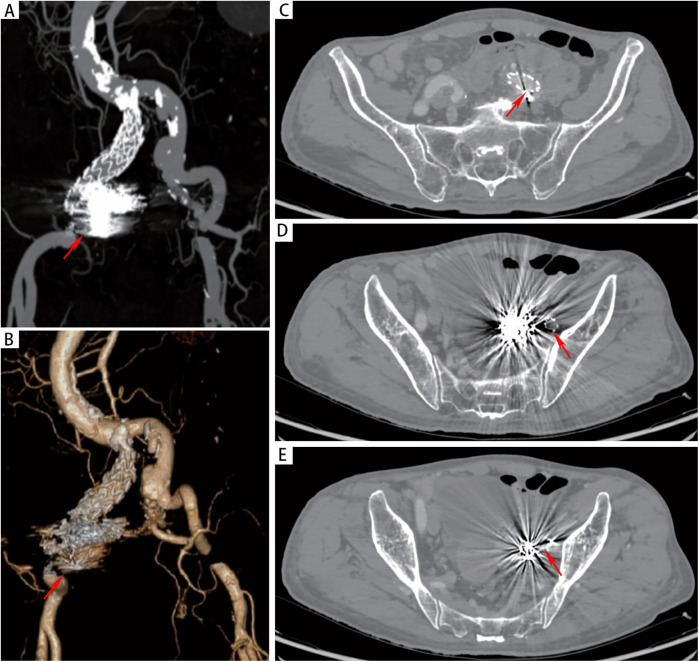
(A,B) CTA at 7 months postoperatively demonstrated patency of the left iliac artery stent and complete occlusion of the left internal iliac artery. (C-E) CT demonstrated absence of gas accumulation in the perigraft region.
